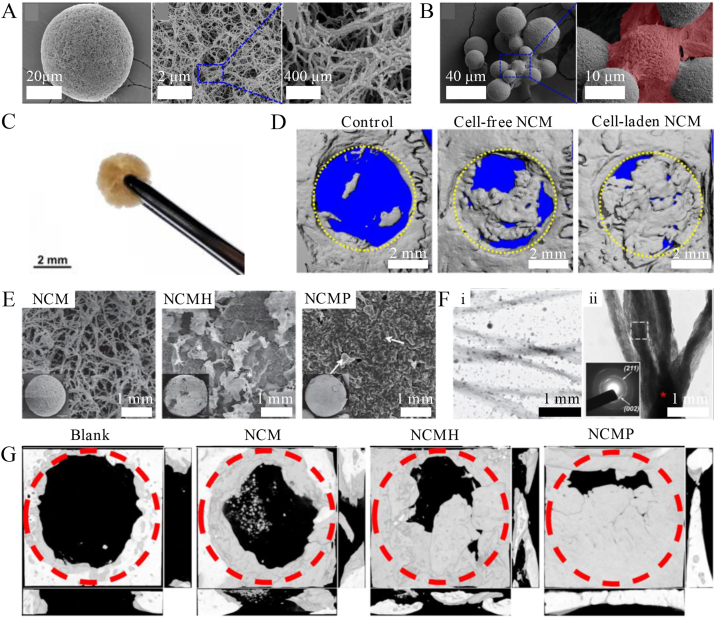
(A) SEM images of NCM. (B) SEM images of MC3T3-E1 cells cultured on NCM after 3 days, cells were rendered in red. (C) Digital photograph of an in vitro macroscopic 3D cell-NCM constructs. (D) Micro CT reconstruction images of bone defect. Reproduced with permission from Ref. [62], Copyright 2022, Elsevier. (E) SEM images of NCM, HAp@NCM (NCMH) and PAH-ACP@NCM (NCMP). (F) TEM images of single-layer collagen with NCMP at 3 days (i) and 5 days (ii). (G) 3D reconstruction images of bone samples treated with NCM, NCMH, and NCMP at 8 weeks. Reproduced with permission from Ref. [63], Copyright 2025, Elsevier. (For interpretation of the references to colour in this figure legend, the reader is referred to the Web version of this article.)
